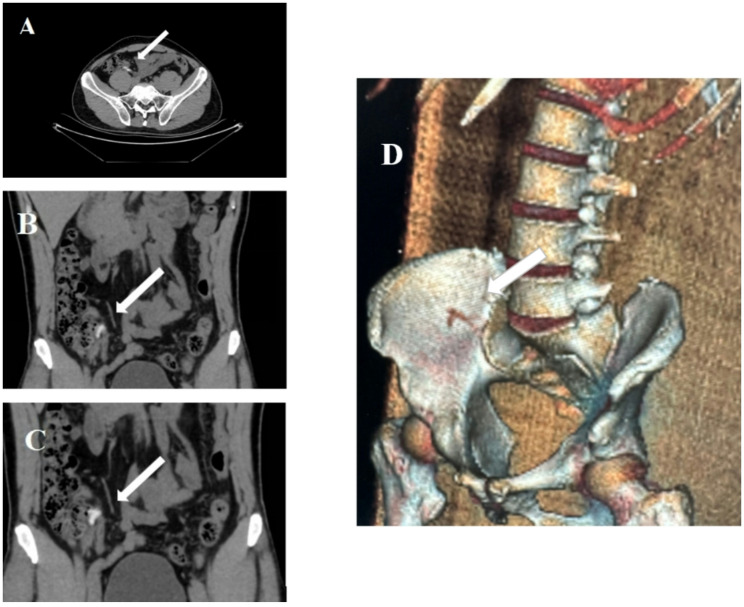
Abdominal CT images and 3D reconstruction showing the suspected Hem-O-Lok clips used during laparoscopic appendectomy (A, axial view; B and C, coronal views; D, 3D reconstruction), with arrows indicating the suspected clips
